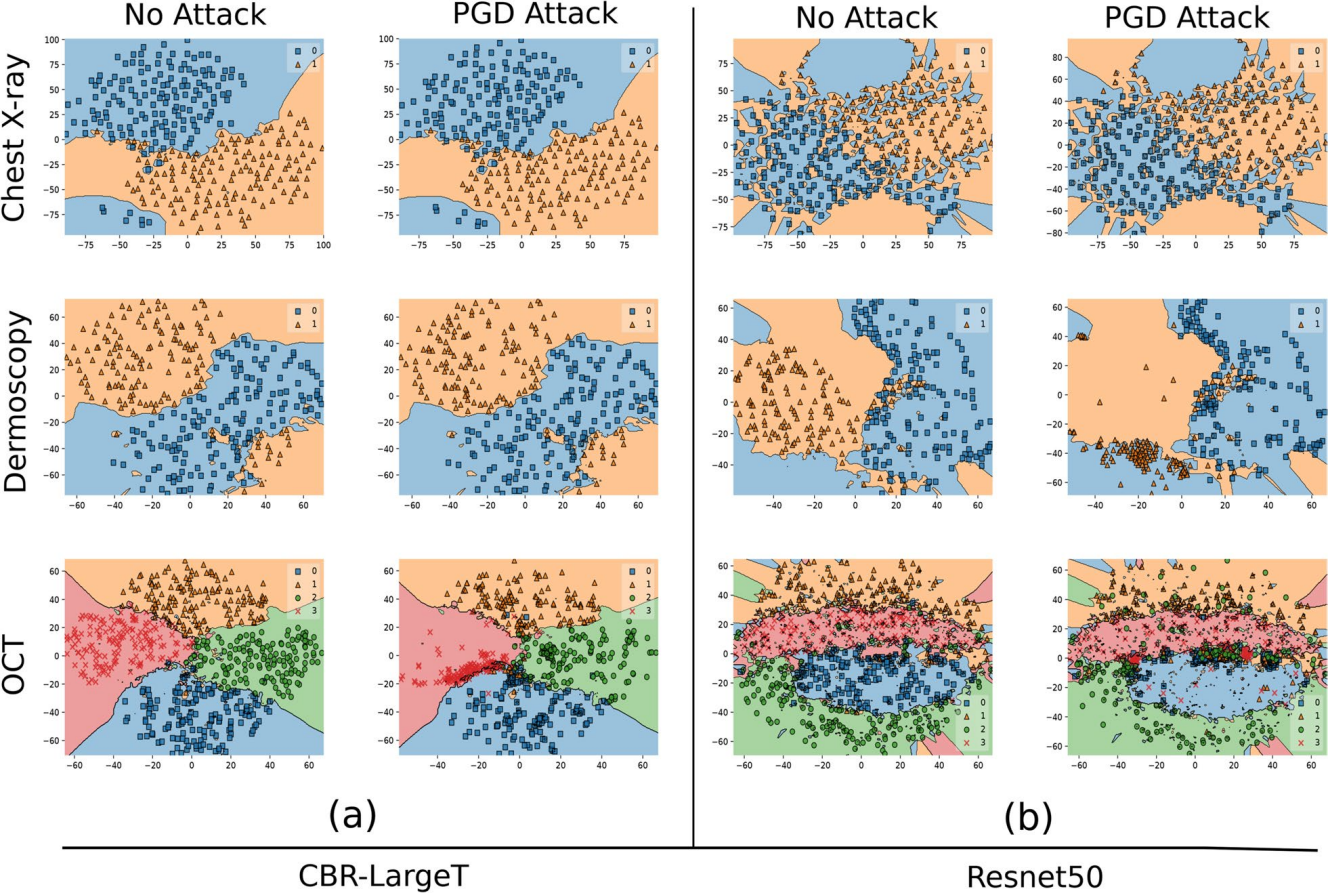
Decision boundary projection of standard trained CBR-LargeT and Resnet50 for Chest X-ray, Dermoscopy & OCT datasets. Unperturbed samples were projected on column 1 (no attack) and perturbed samples were projected on column 2 (PGD attack) for (a, b), respectively. The more complex decision boundary in (b) resulted in samples that were closer to the decision boundary in the projected space which increased medical image DNN sensitivity to input perturbations
